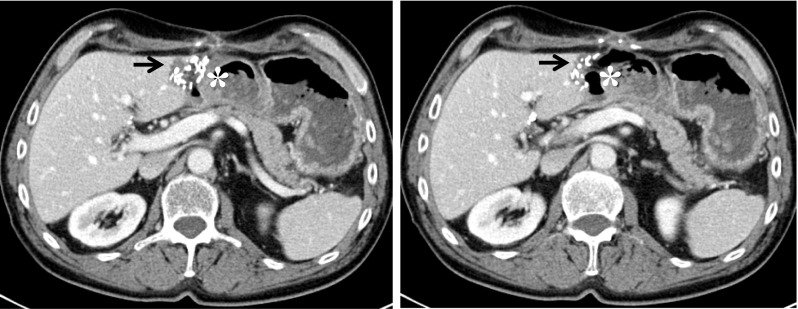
Two subsequent CT images of a patient with tumor recurrence at the left hepatic resection margin (arrows). The air-filled colon transversum lies directly adjacent to the clips of the resection margin (asterisk)
